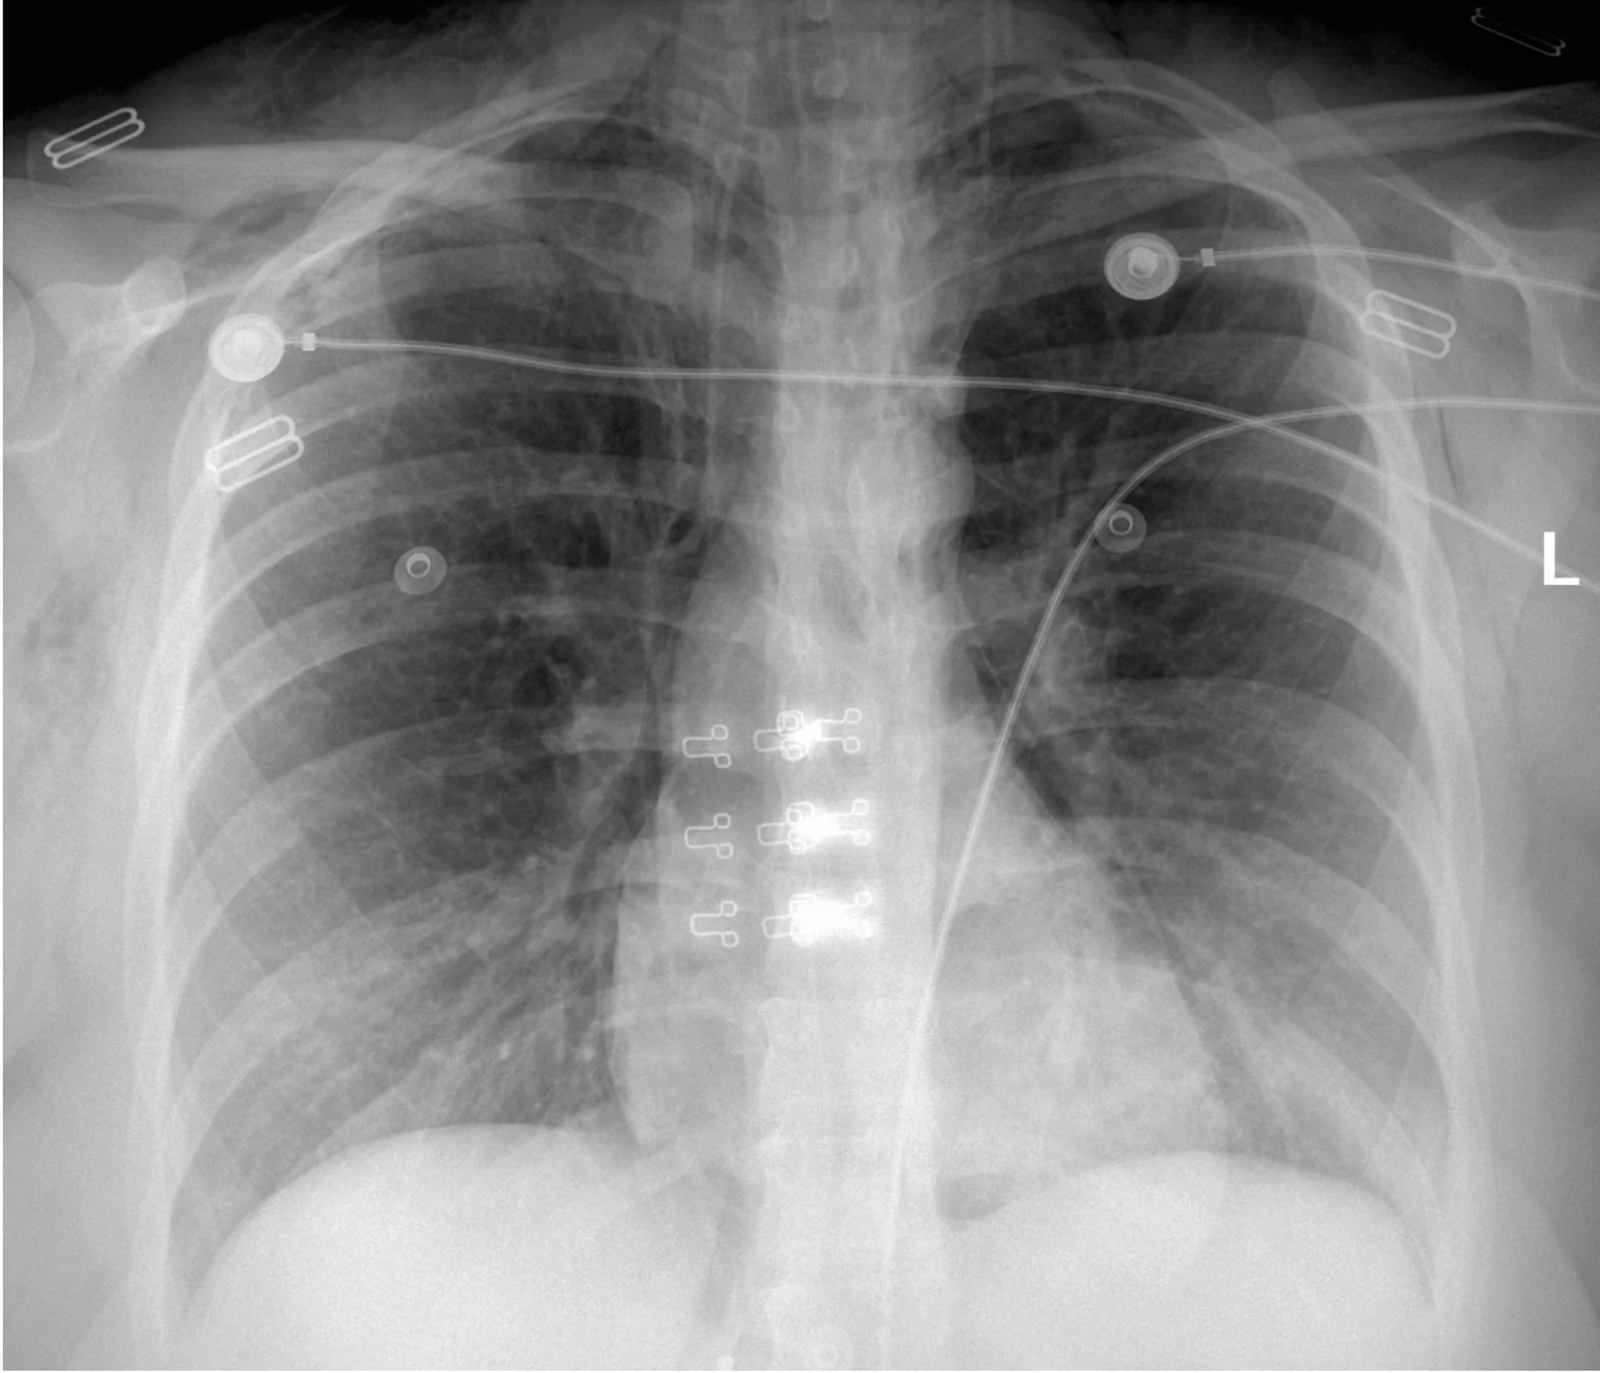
X-ray of the chest AP view showing subcutaneous gas across the lower neck associated with pneumomediastinum and pneumopericardium.
